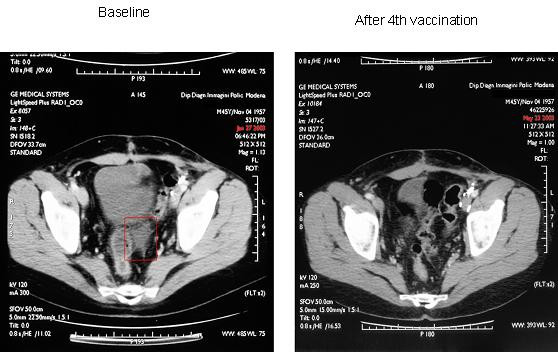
Baseline and post 4^th ^vaccination CT scan of patient no. 12, treated with mDC. The patient had an 8-month CR of the abdominal soft tissue lesions.
